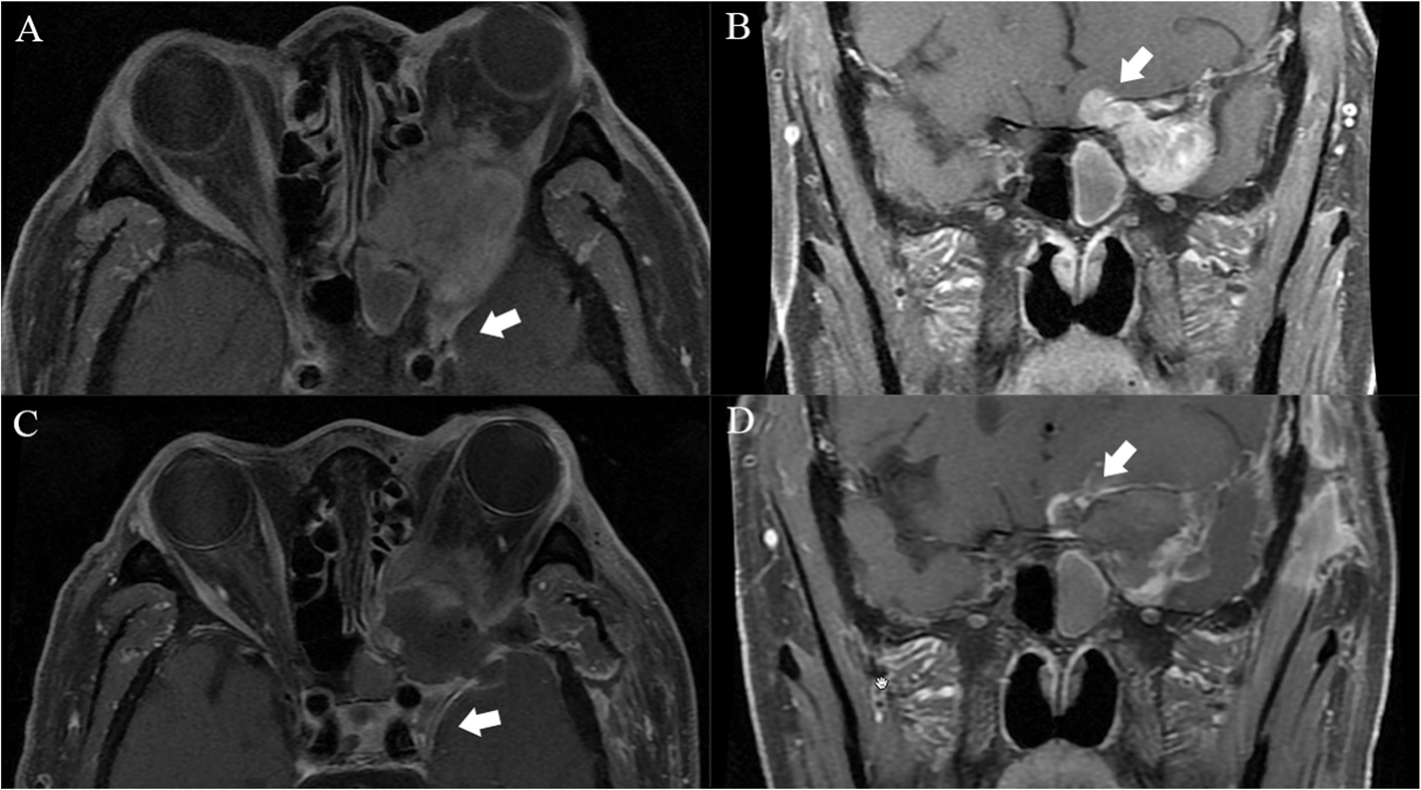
Case 3. The patient was a 78-year-old with a chronic disease process, left exophthalmos, and vision loss for more than 19 years. a: MRI showed that the main body of the tumor was located lateral orbit and invaded the intracranial dura, and cavernous sinus through the superior orbital fissure (arrow). b: The tumor broke through the dura mater of the skull base and invaded the intracranial structure (arrow). c, d: After the Transfronto-orbital approach was given to the craniotomy, the MRI re-examinations immediately after the operation showed that the tumors involving the cavernous sinus and intracranial were removed completely. There was no recurrence after 3 years of MRI follow-up
